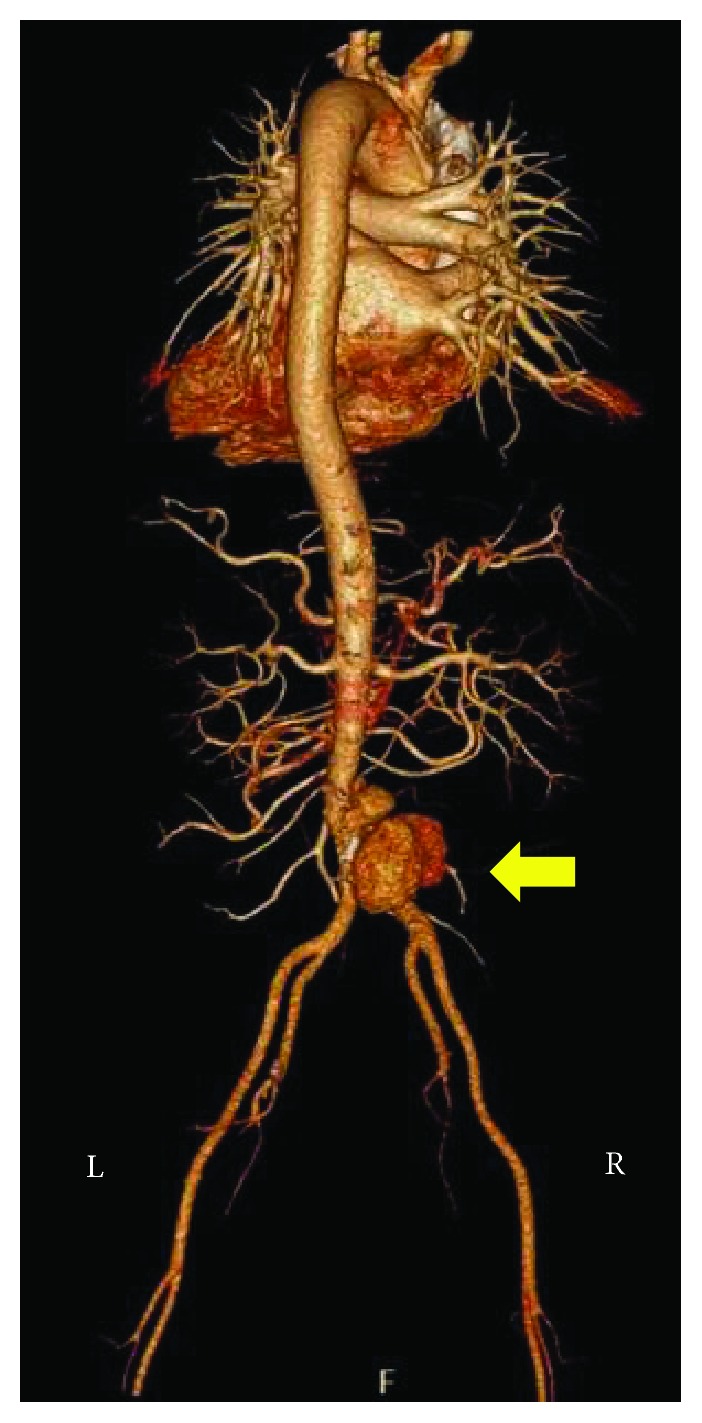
This is a 3-dimensional reconstruction of the CT aortogram, with the arrow indicating the saccular component of the aneurysm.
